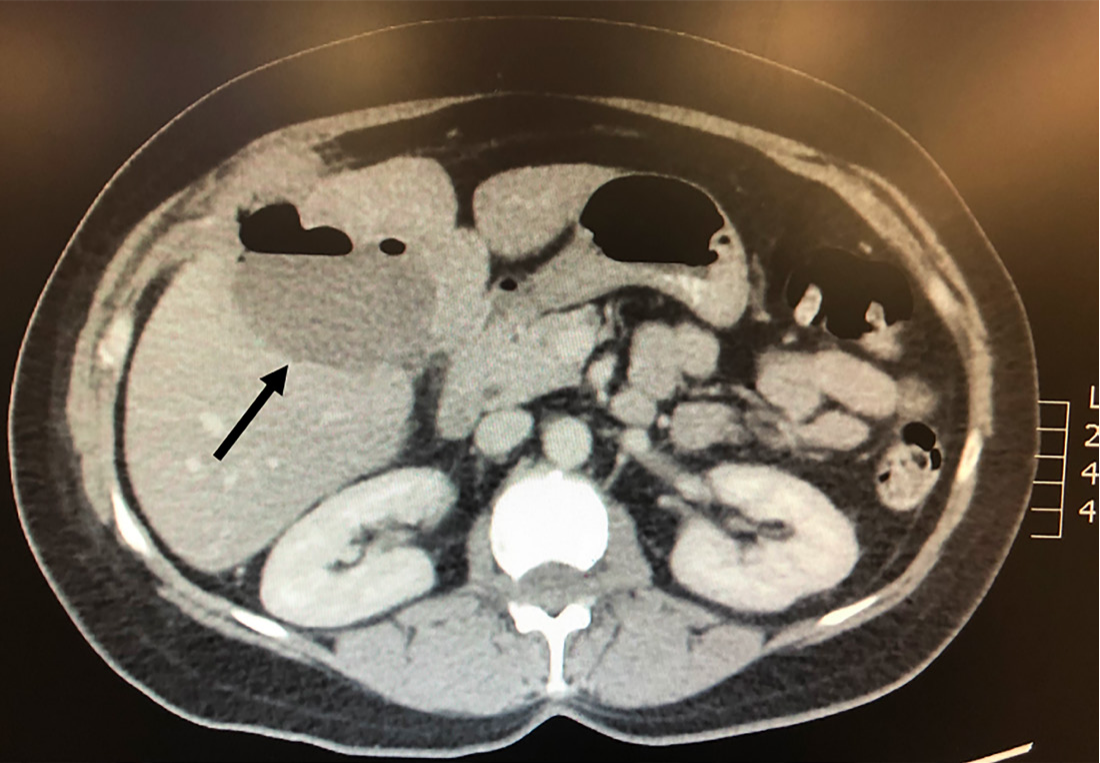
Transverse view of computed tomography of abdomen/pelvis with intravenous contrast demonstrating a 7.3 × 5.7 × 7.7 centimeter rim-enhancing fluid collection with air-fluid level in the gallbladder fossa, denoted by black arrow.
